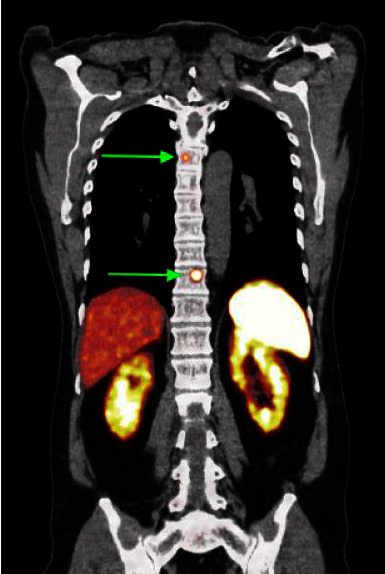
PET-CT 68Ga-DOTATATE scan showing paravertebral lesions (arrows).
